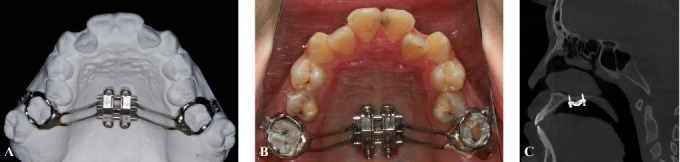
The use of MSE. (A) The structure of MSE. (B) Placement of MSE. (C) The sagittal plane in CBCT and mini-implants passed through the double cortex of the maxilla.
